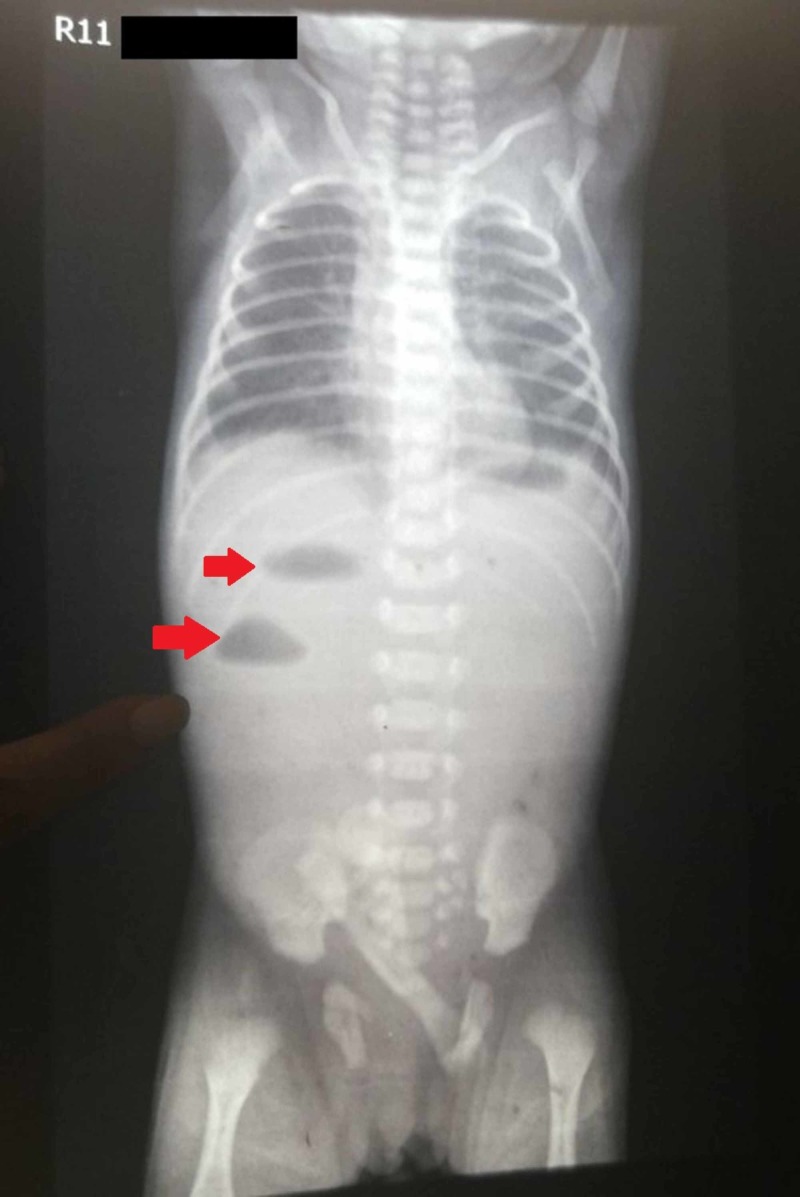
X-ray abdomen showing two air-fluid levels in the left hypochondrium
